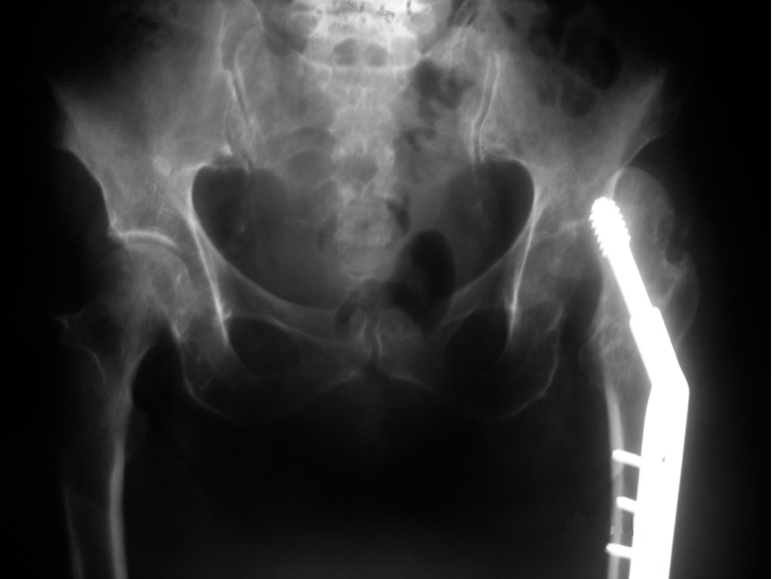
X-ray demonstrating a posterior hip dislocation while the old trochanteric fracture has healed.
